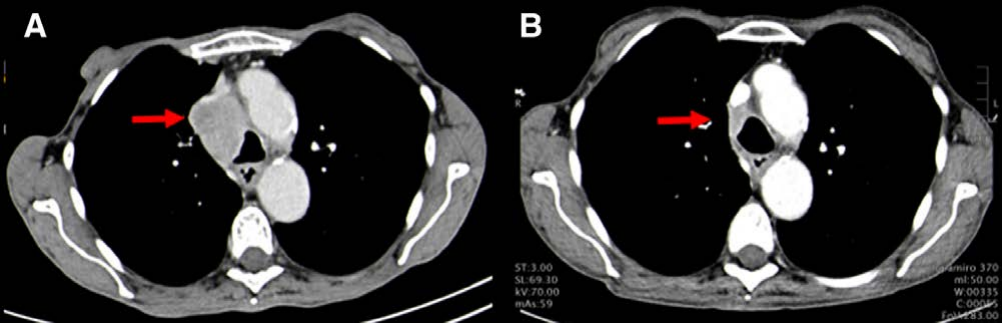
Radiologic response to selpercatinib. (A) CT scan of the chest post 4 cycles of chemotherapy and prior to selpercatinib initiation, revealing a large mediastinal lymph node in position 4R (red arrow). (B) Follow-up CT scan of the chest showing substantial lymph node regression (red arrow) after 2 months of neoadjuvant selpercatinib treatment. CT = computed tomography.
